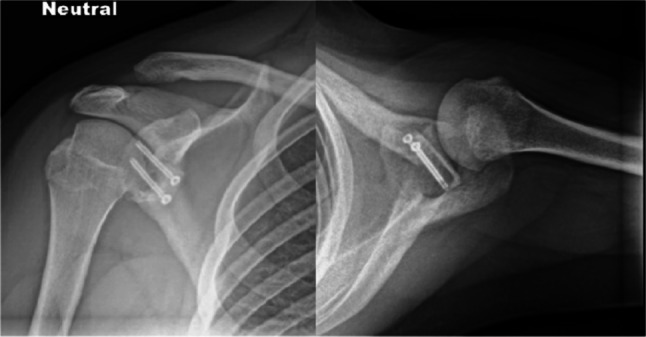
Post-operative radiographs following a Latarjet procedure for a 15-year-old adolescent athlete with recurrent shoulder instability and glenoid bone loss following prior arthroscopic Bankart repair. Figure taken from Kay et al. 2023 [25]. Reprinted with permission from Springer Nature [25]
